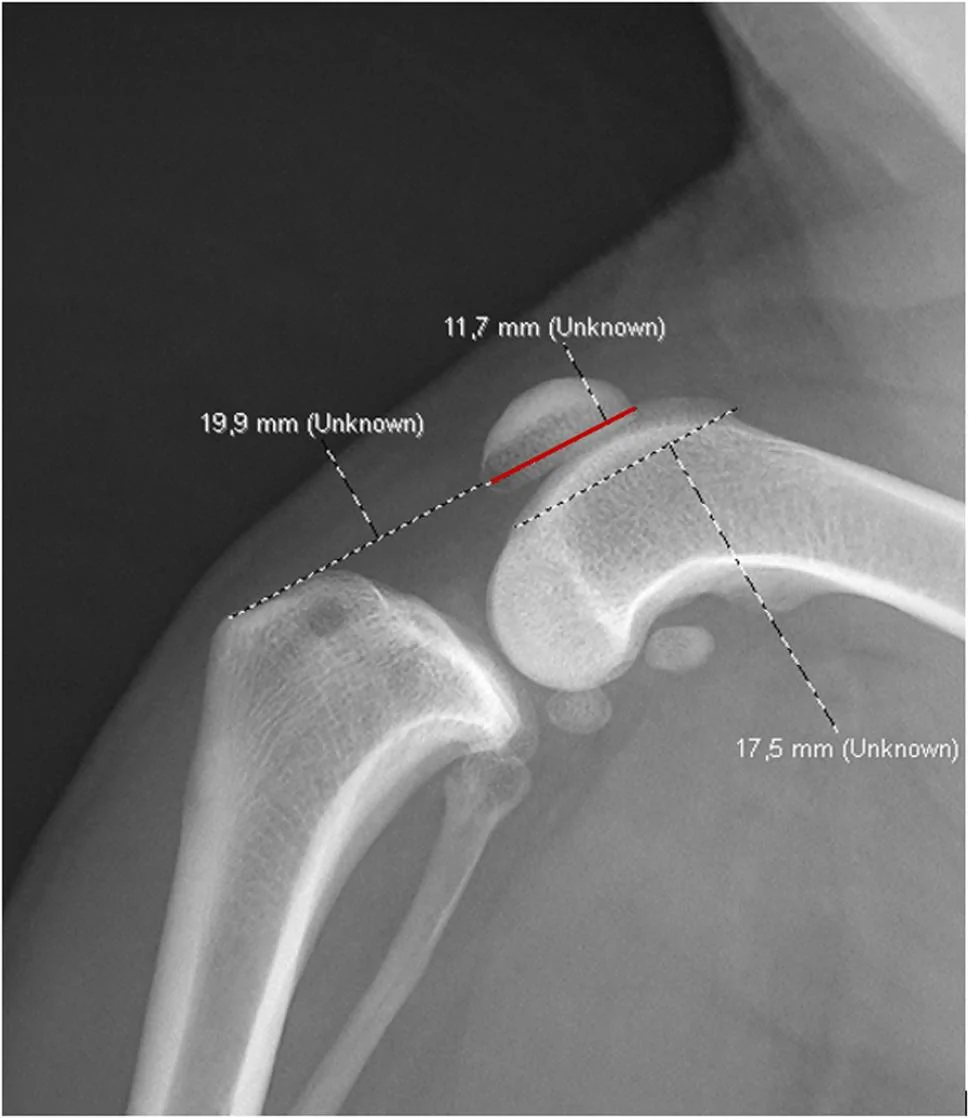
Morphometric measurements on medio-lateral radiographs, determining the patellar length (PL, marked in red), the length of the trochlea (TL) and the patellar ligament length (PLL)
